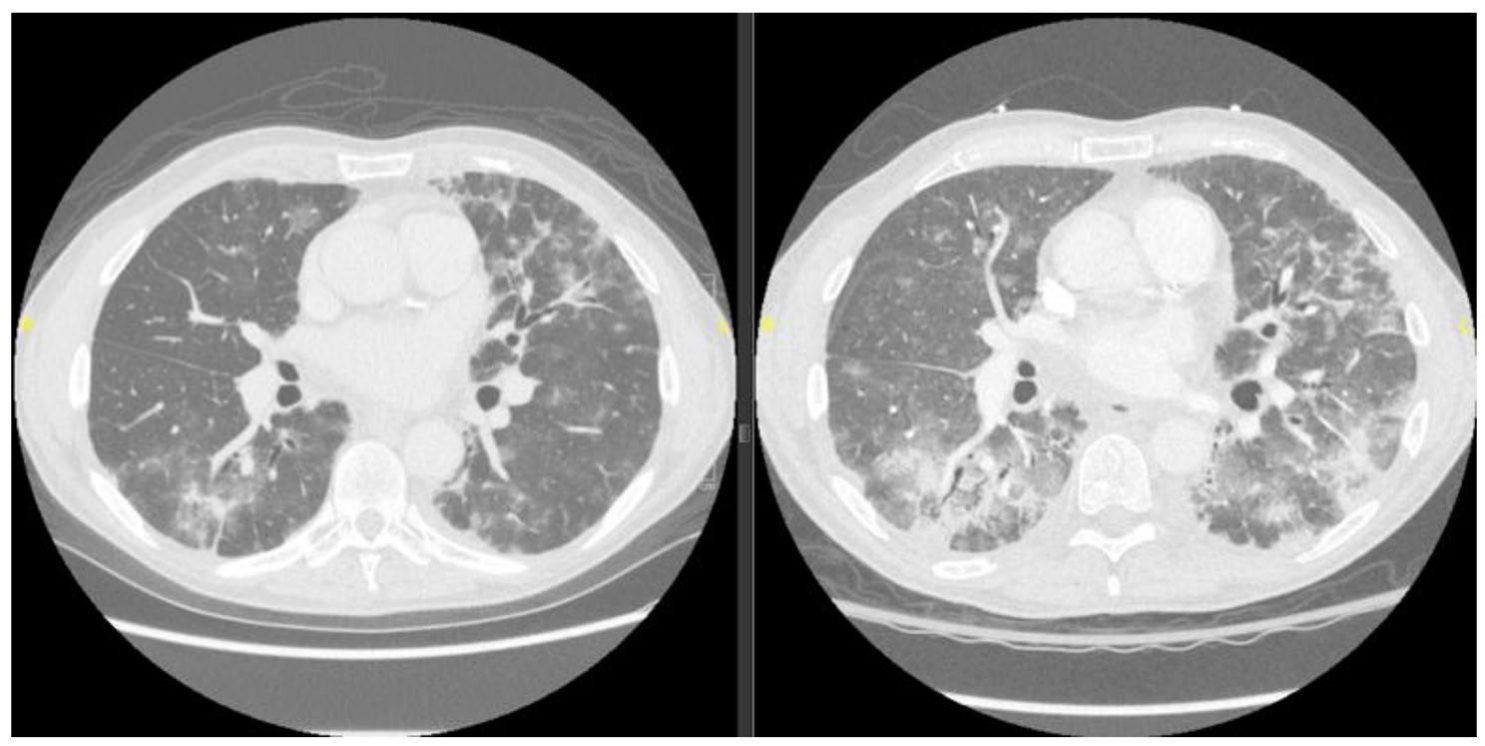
Chest CT scan at clinic (left) and at hospital admission 11 days later (right)
